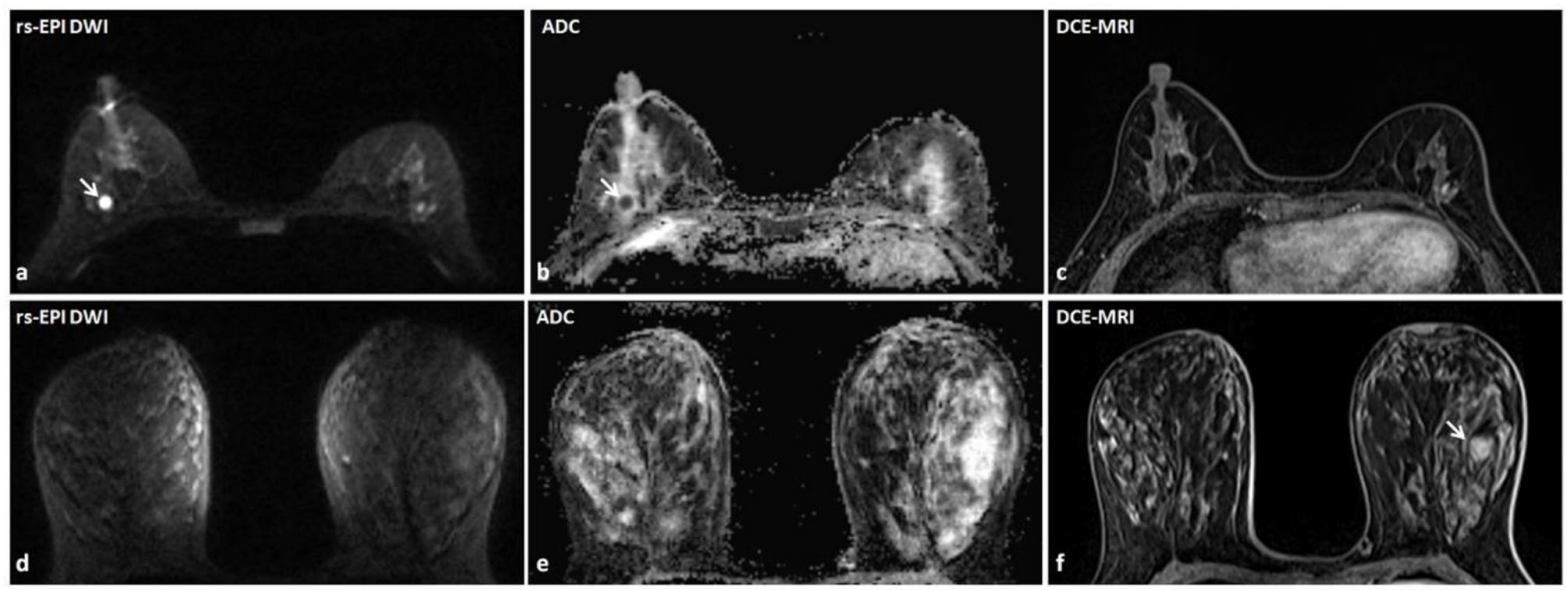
Discrepant findings of DCE-MRI and rs-EPI DWI for detecting breast lesions. (a) rs-EPI DWI (*b*-value, 1,000 s/mm^2^) shows a round, well-defined, and homogeneous nodule (6.7 mm) (arrow) with markedly low signal on ADC map (b) (mean ADC value, 0.47 × 10^−3^ mm^2^/s) (arrow) in the right breast of a 43-year-old woman, whereas (c) DCE-MRI shows no abnormal enhancement at that location. This lesion was pathologically verified as the right breast fibroadenosis. (d–f) rs-EPI DWI (*b*-value, 1,000 s/mm^2^), ADC map, and DCE-MRI of a 47-year-old woman with the left breast adenosis. (f) DCE-MRI depicts a well-defined lesion (14.6 mm) (arrow), whereas there is no abnormal signal on rs-EPI DWI (d) and ADC map (e).
